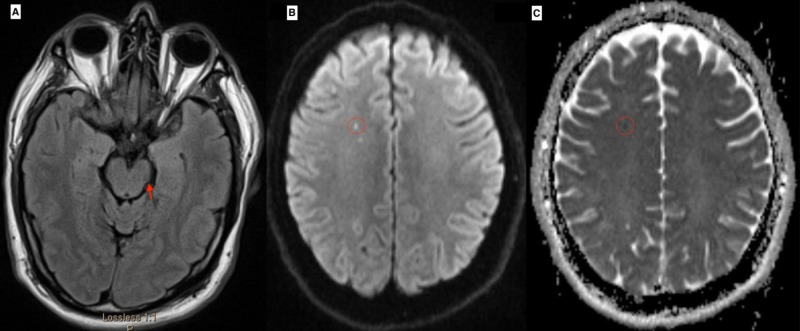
Initial magnetic resonance imaging (MRI) of the brain on axial sequence at the level of the midbrain shows a lacune (arrow) on T2 fluid attenuated inversion recovery (FLAIR), suggestive of an old infarct (1A). On follow-up one month later, a tiny punctuate infarct at the level of the centrum semiovale in the right frontal region (1B) (circle) with the corresponding apparent diffusion coefficient (ADC) correlate (1C) (circle) is seen.
